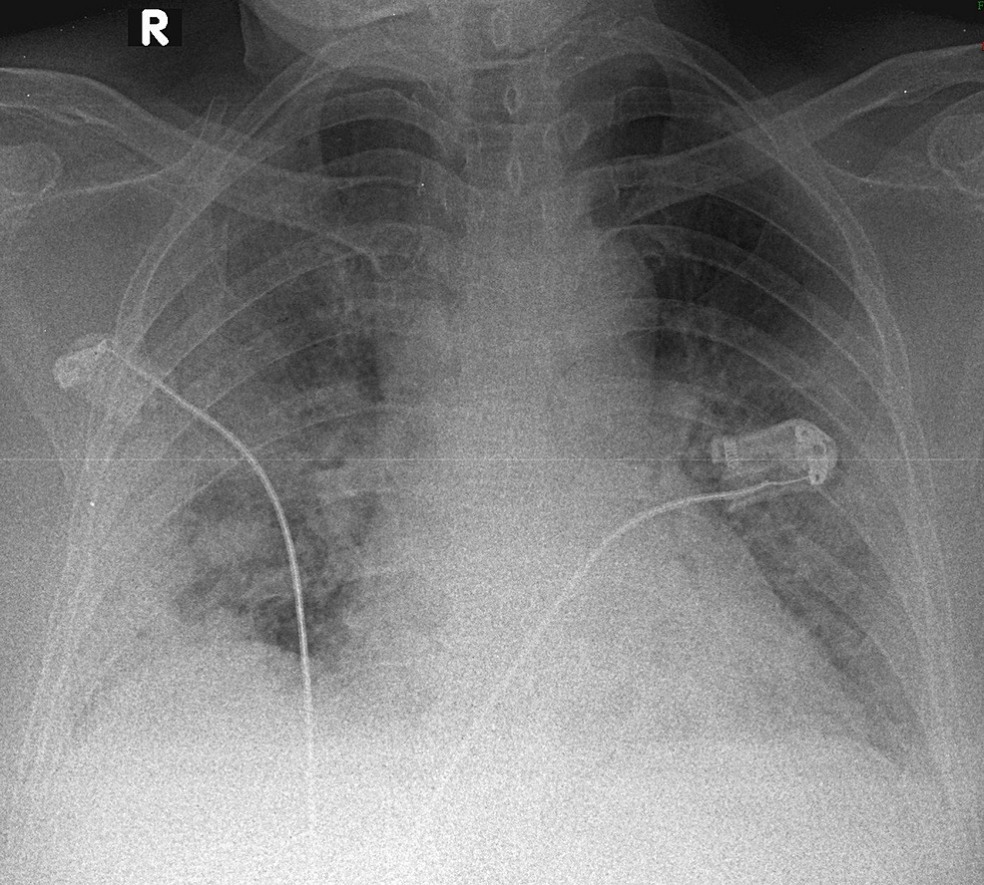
Typical radiographic pattern in a critical case of SARS-CoV-2 pneumonia This CXR showed ground-glass opacities and consolidations in bilateral, peripheral lower, and mid-zones. The Brixia score was 12, the RALE score was 7, and the mCXR score was 9. This case was classified as a critical situation based on clinical severity. The qCSI was 9.
